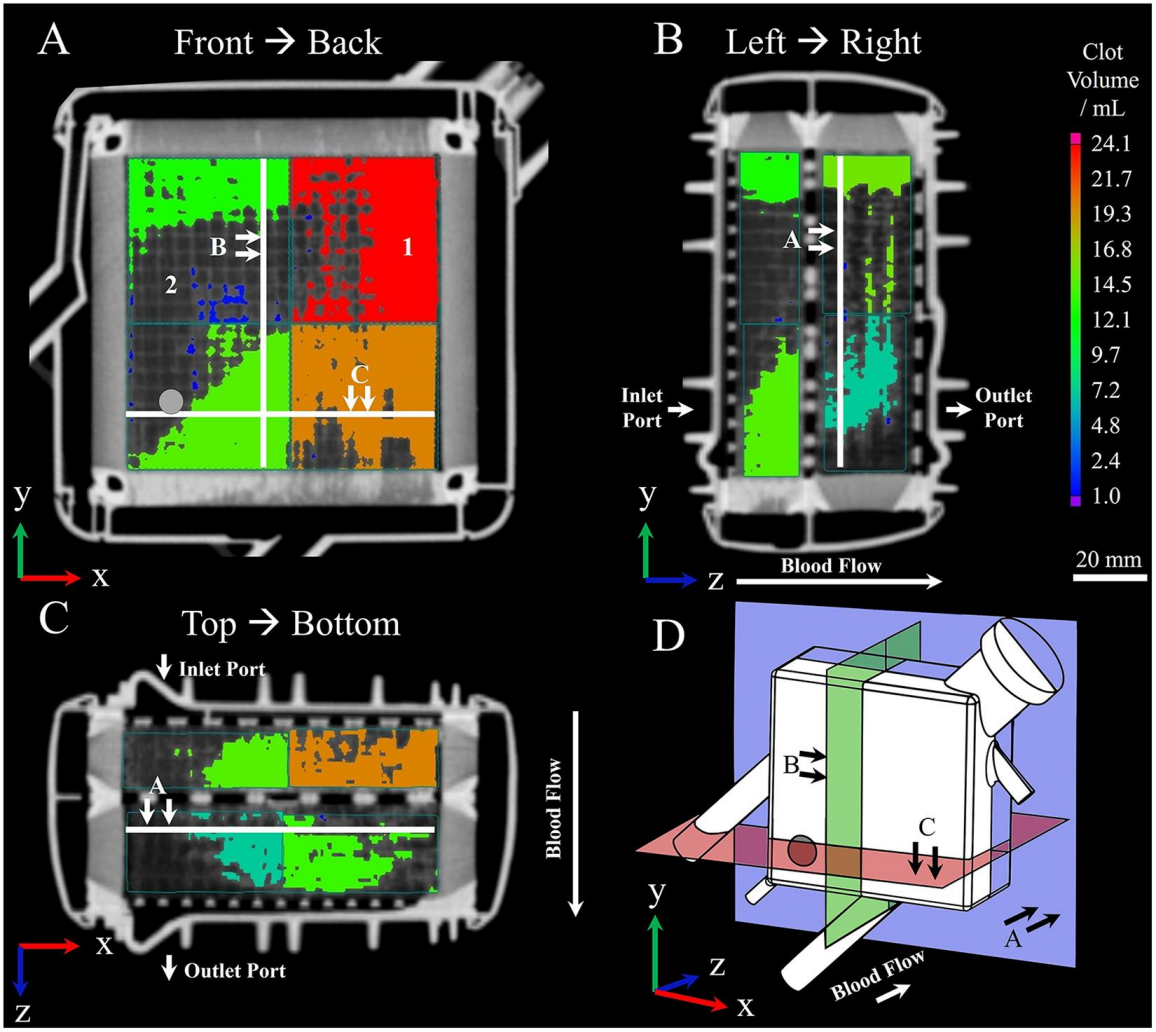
Visualization of (1) clotted and (2) clot-free areas within MLs prior to embedding using clinical multidetector computed tomography; procedure according to Wagner et al. (20). Clot depositions are shown in color. Wagner et al. (20) virtually divided the ML into eight sectors and calculated the contained clot volume within by applying a threshold-based algorithm. The resulting clot volume is color-coded and corresponds to the color bar located on the right-hand side. (A) Front to back perspective; gray circle indicating position of inlet port. (B) Left to right perspective. (C) Top to bottom perspective. (D) Three-dimensional representation of displayed planes.
